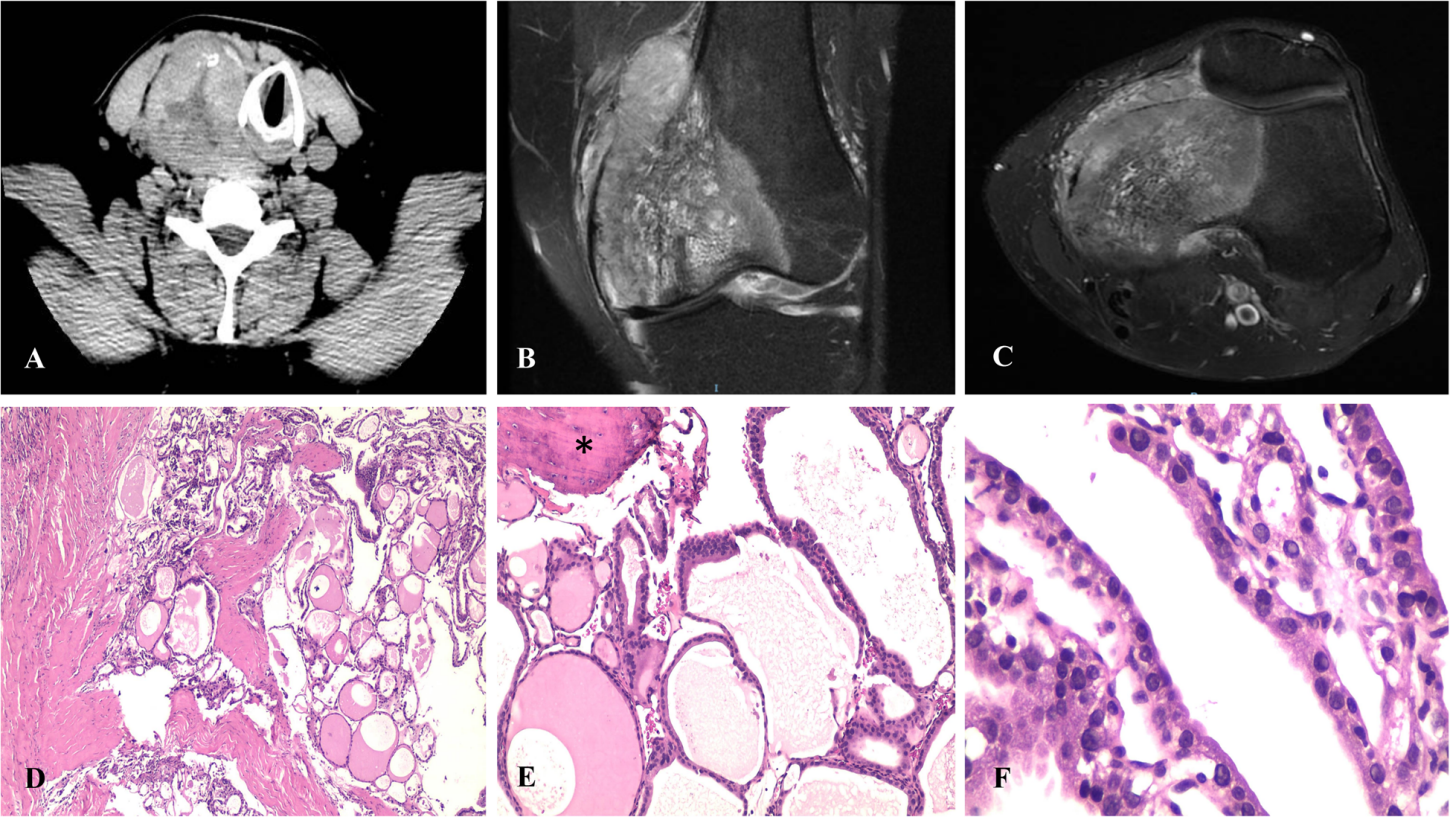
Metastatic macrofollicular thyroid carcinoma to femur. a CT showed the presence of a tumor involving right thyroid lobe with central necrosis that displaced the upper third of the trachea and the vascular structures on the right side of the neck; b and c MRI showed a destructive neoplasm in the diaphysis and epiphysis of the femur; d The neoplasm showed large follicles in more than 50% of the neoplastic follicles; e Immersed in the metastasis of macrofollicular variant papillary thryroid carcinoma, a bone spicule was observed (*); f Follicles were lined by atypical nuclei and some showed pseudoinclusions
